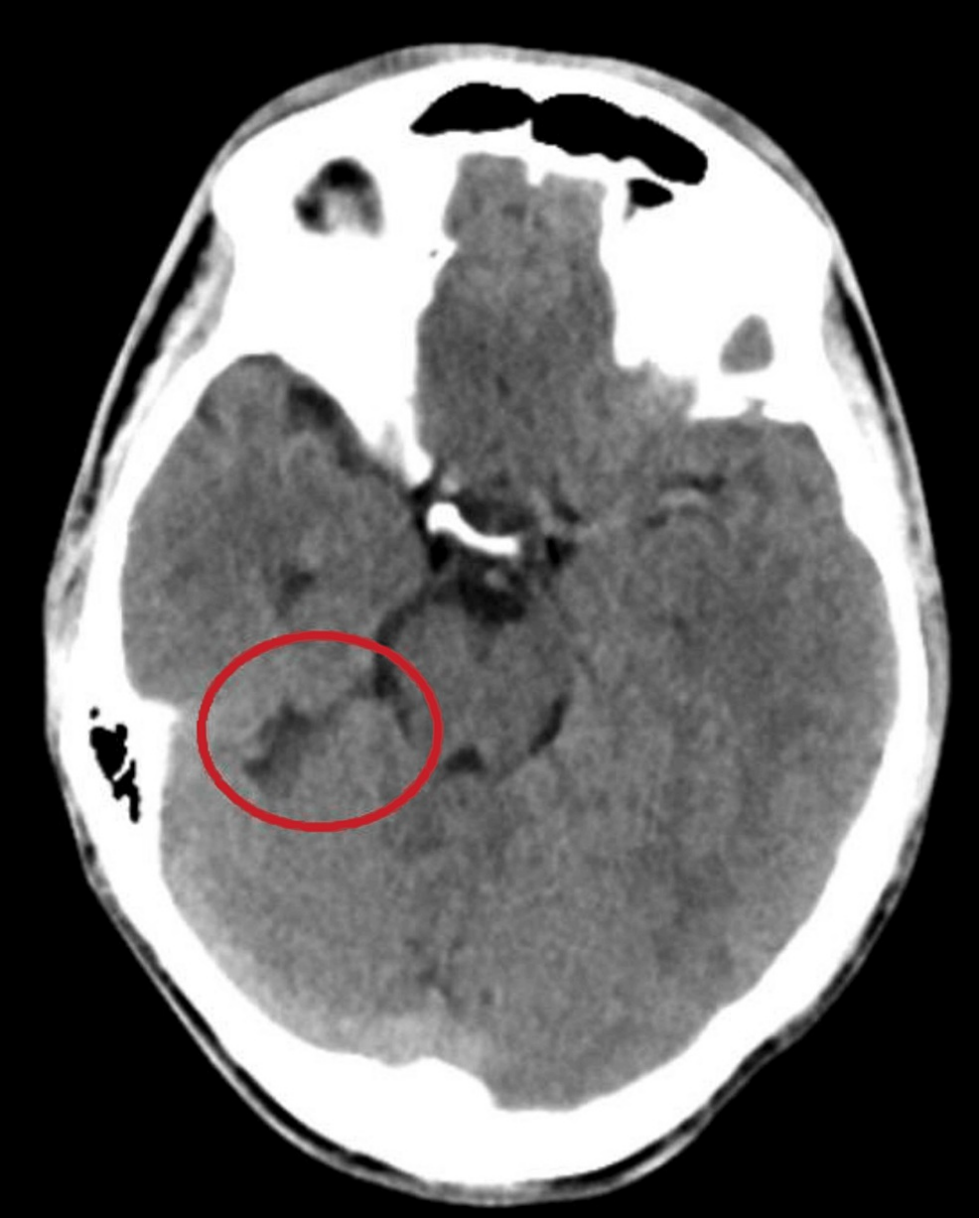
CT head without contrast demonstrating right temporal lobe closed-lip schizencephaly
